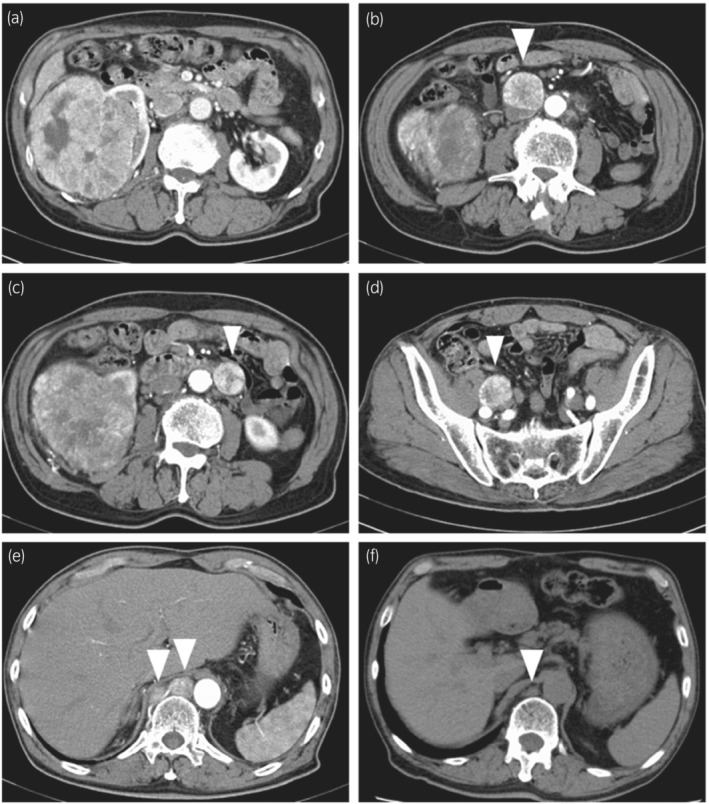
Abdominal contrast‐enhanced computed tomography at the initial diagnosis. Imaging revealed (a) a 12‐cm renal tumor in the right kidney and multinodal metastases in the (b) inter‐aortocaval, (c) para‐aortic, (d) external iliac, and (e) posterior mediastinal regions. (f) Abdominal plain computed tomography 1 year after administration of axitinib shows shrinkage of the posterior mediastinal lesions.
